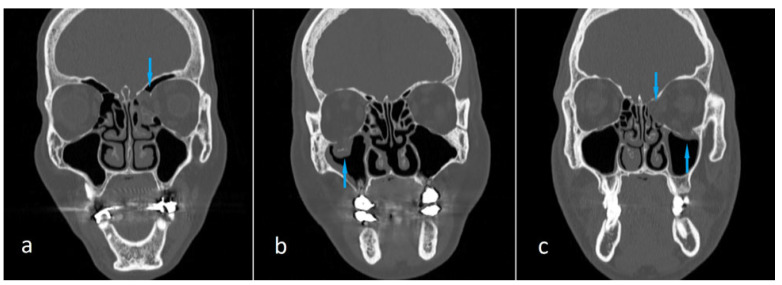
Coronal maxillofacial computed tomography images showing orbital blowout fractures located in the medial wall (a), orbital floor (b), and both the medial wall and orbital floor (c) (arrows).
